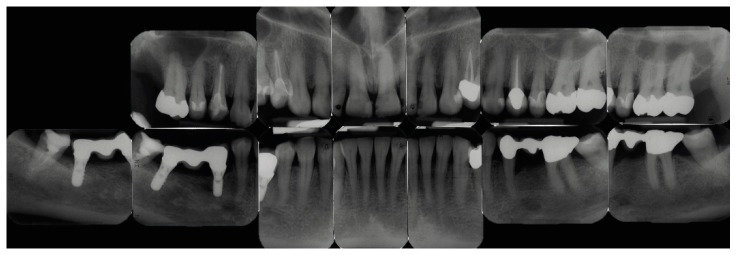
Full-mouth intra-oral radiographic set (I-O) represented by periapical films.
